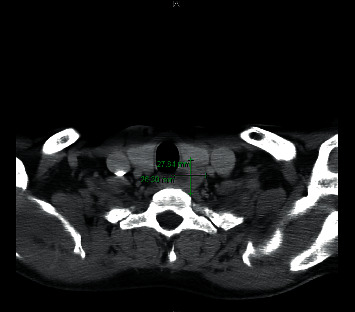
CT neck, transverse plane. A 2.8 × 2.6 cm complex nodule in the left lobe of the thyroid. On further examination by way of a thyroid ultrasound, the lesion was further characterized and described as an echo poor mass showing lobulated margins and coarse internal calcification, highly suspicious for malignancy.
